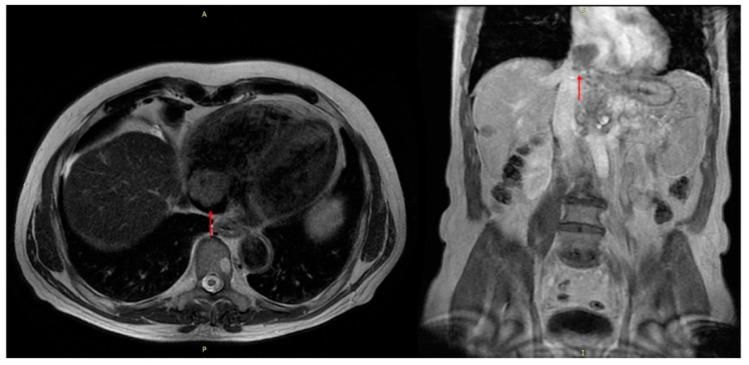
CT scan in patients with hepatocarcinoma. Red arrow: extensive thrombosis, originating in the hepatic veins (not visible in these scans), invading the right atrium through the inferior vena cava.
